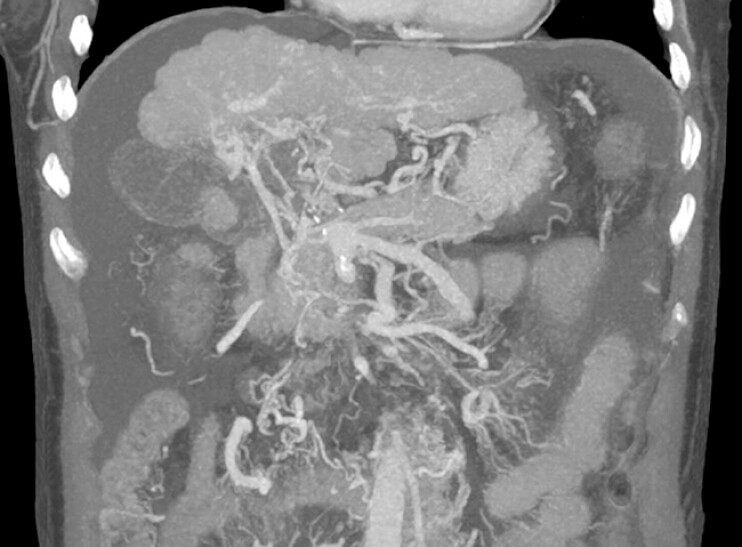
CT venography showed liver cirrhosis with PC and ascites. CT, computed tomography.
